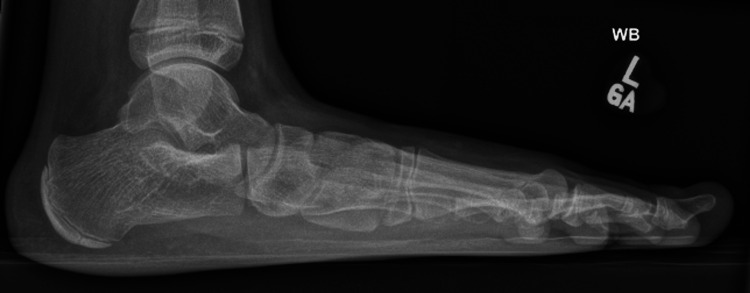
A pre-operative weight-bearing lateral radiograph of the left foot demonstrating a low calcaneal pitch and negative Meary's angle
